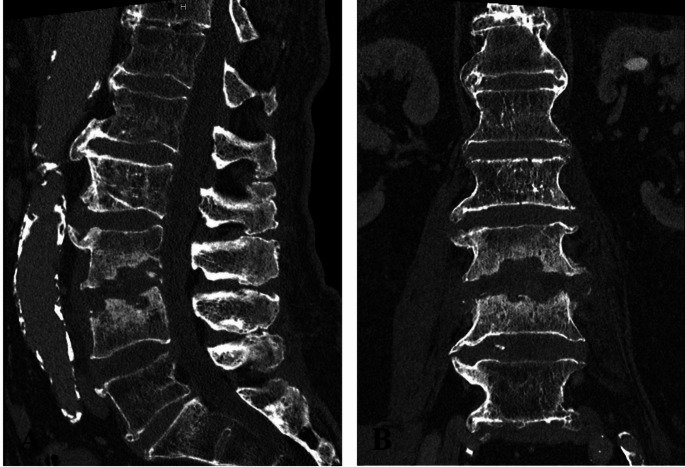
Preoperative CT-Images show destructive spondylodiscitis L3/L4 (A: sagittal plane; B: frontal plane)
